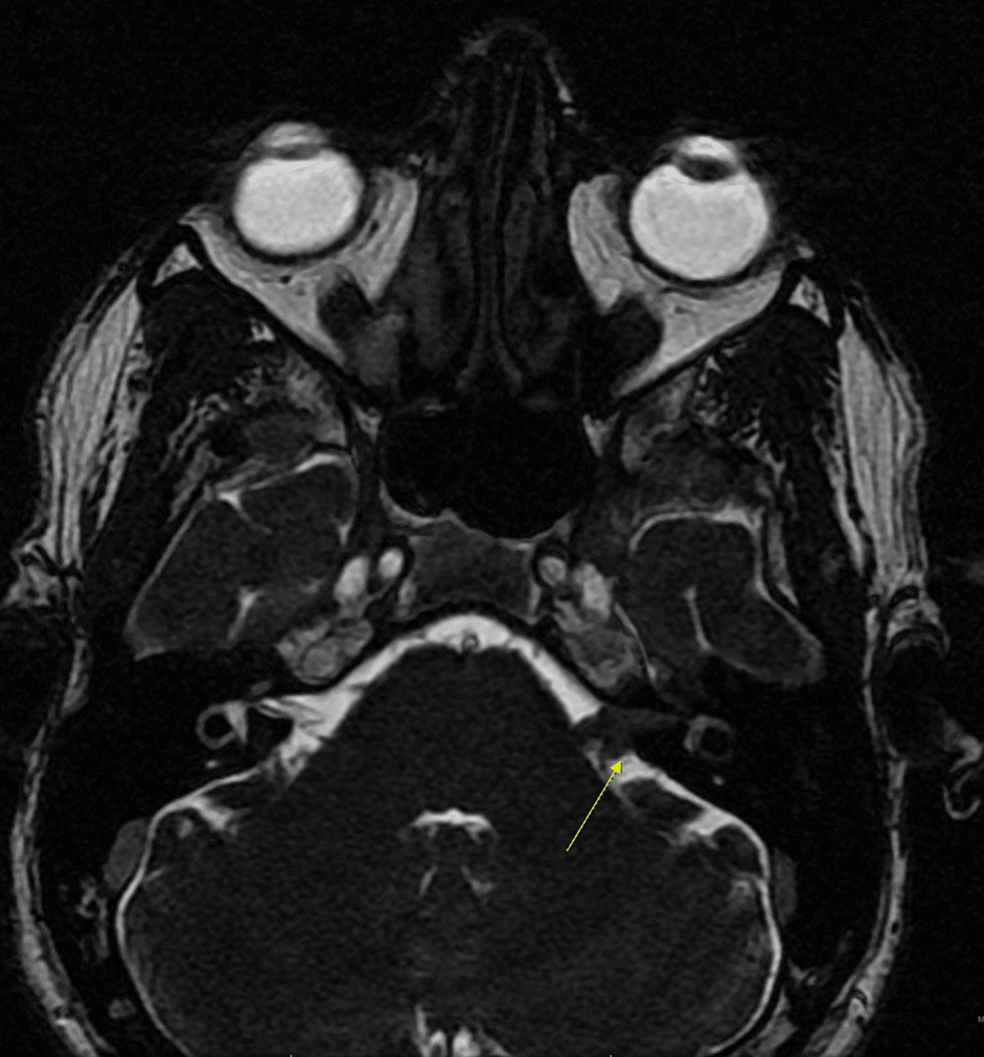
Initial axial T2 heavy-weighted (FIESTA/CISS) sequence MRI of the brain The image shows an abnormally decreased signal in the left internal auditory canal and inner ear structures (arrow). CISS - Constructive Interference Steady State
